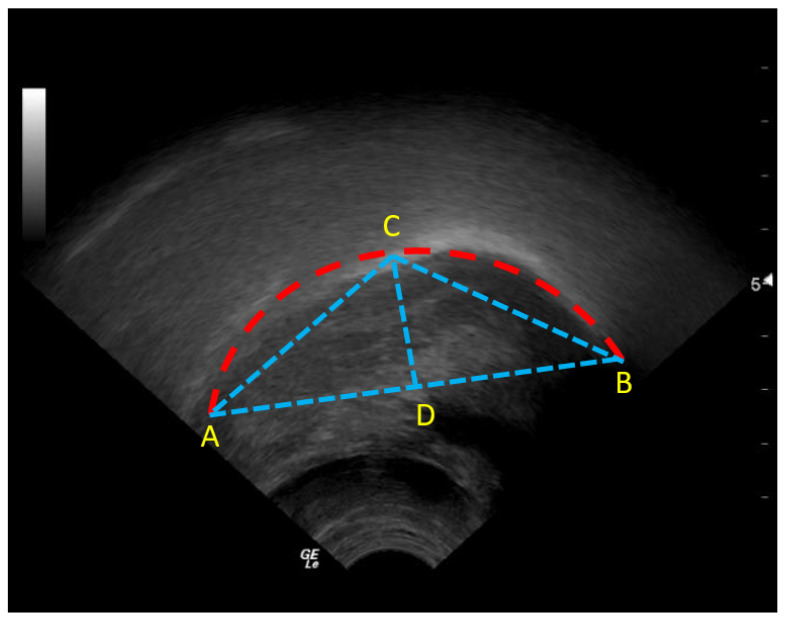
Shape-based evaluation measure. Point (A) is on the dorsal tongue part, point (B) is the point on the tongue tip, point (C) is the apex. Point (D) is the projection of point (C) on the (AB) line. The copyright for this ultrasound picture belongs to the author of this article, Khalid Al-hammuri [5].
